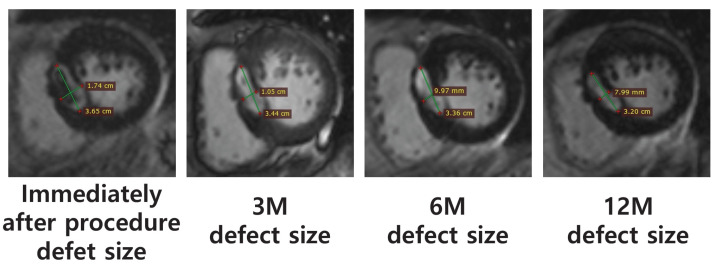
Changes in defect size over the follow-up period, based on MRI measurements.
